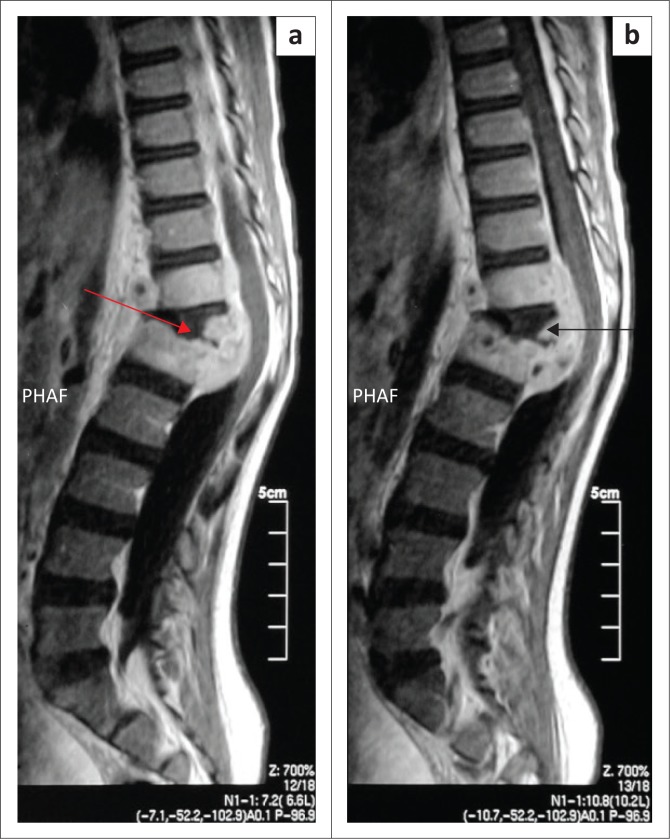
The magnetic resonance imaging of the spine revealed (a) kyphotic deformity and (b) pre-vertebral fluid collection at T12/L1 extending to the epidural space.
